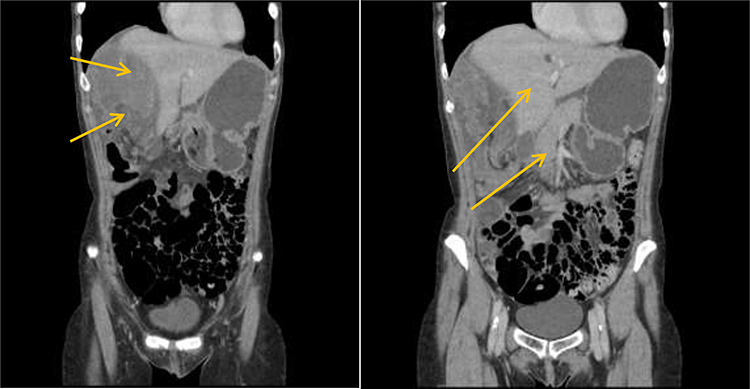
CT scan shows interposition of ileum, cecum and ascending colon between liver and diaphragm pushing right loop of the liver and gallbladder medially.
